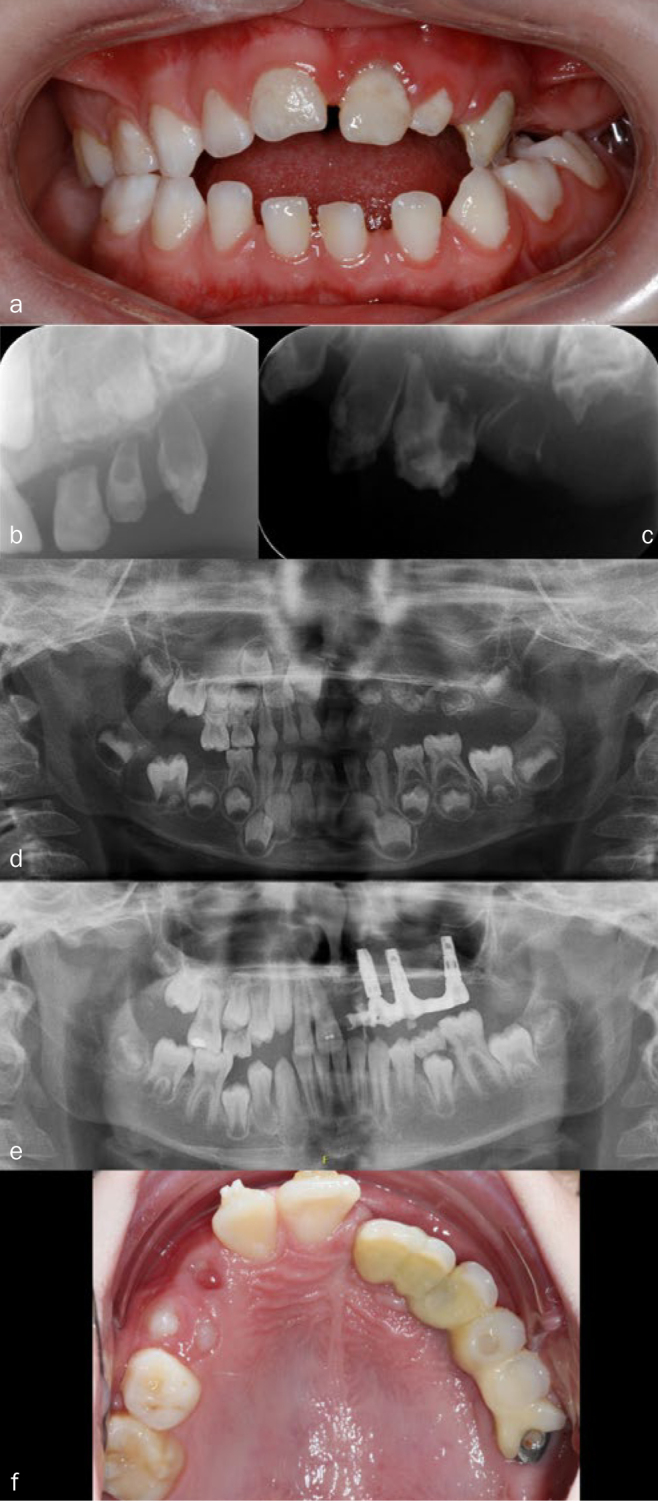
Treatment of patient C, followed-up for 6 years (from 4.5 to 10 years of age). a) Clinical photo at age 4.5 years. b) + c) Radiograph at age 4. d) Orthopantomogram at age 4.5. RO was seen on the maxillary left side affecting 61, 62, 63, 64, 65, 21, 22, 23, 24, 25, and 26. In addition, a cyst was seen in region 46. 75 initially in supraposition. e) Radiograph taken at age 9 (3 years after surgery) shows three implants in the RO affected region. Cyst gone and 46 erupted normally. f) Clinical photo of teeth at age 9. Note the shift of the midline.
